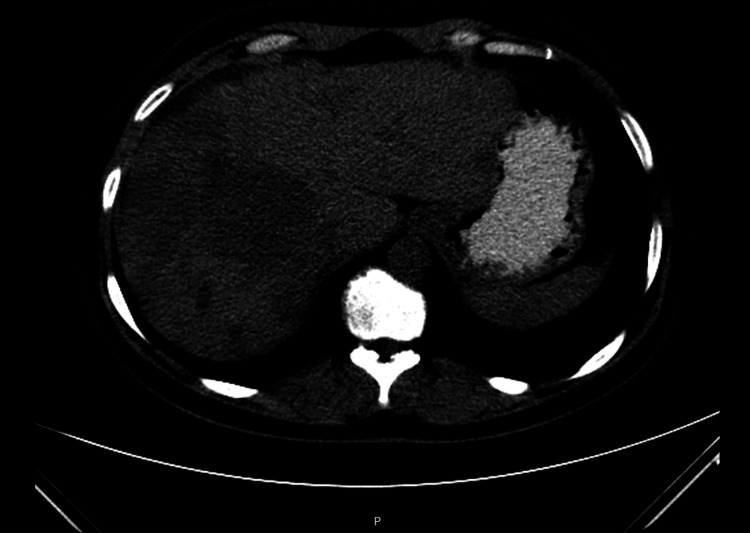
Axial CT image in March 2016 showing diffuse hepatic progression.
